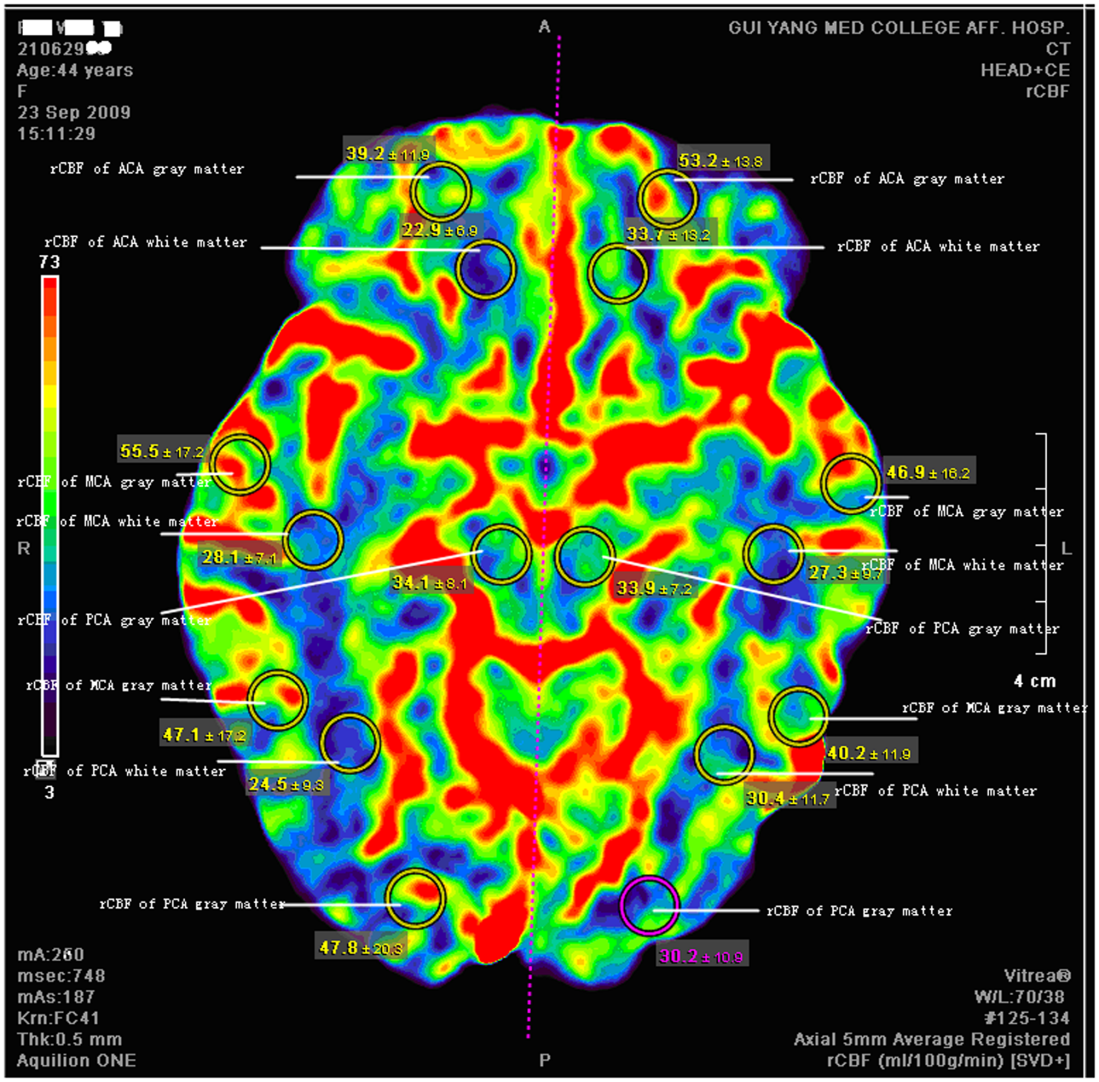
Case example: Coronal slices from dynamic CT angiography taken from a 44-year-old woman with depression. Circles represent regions of interest, with the mean ± SEM from 19 volume data presented. In MCA (middle cerebral artery) regions, the values of rCBF (regional cerebral blood flow) in left gray matter were lower than in the right [Left: 46.9 and 40.2 ml/(min·100 g),Right: 55.5 and 47.1 ml/(min·100 g)]. In PCA (posterior cerebral artery) regions, the values of rCBF in left gray matter were lower than in the right [Left: 30.2 ml/(min·100 g), Right: 47.8 ml/(min·100 g)].
